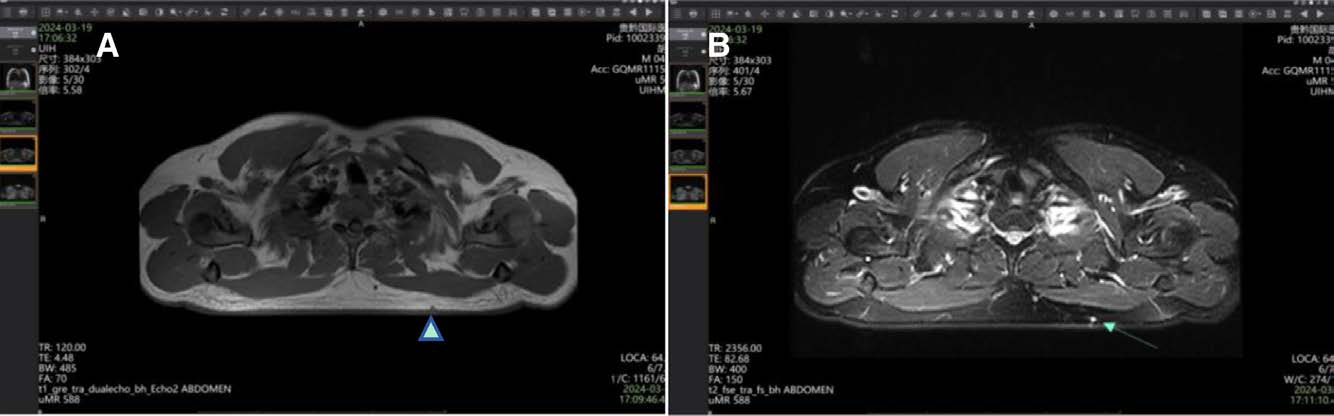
MRI revealed a small, abnormal signal measuring approximately 3.1 × 3.0 mm located beneath the subcutaneous fatty tissue in the left scapular region. The images displayed T1 hypointensity (A) and T2 hyperintensity (B), with a clear boundary observed around the lesion. MRI = magnetic resonance imaging.
